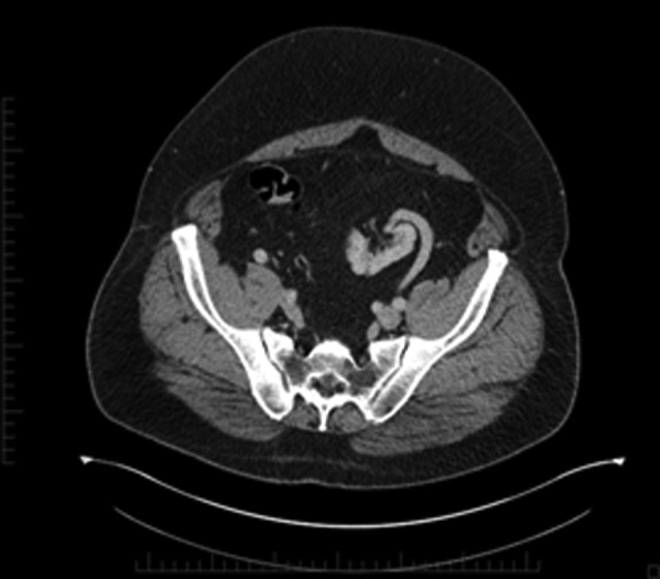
Case 1—CT urogram; axial image showing the medial location of a congenital pelvic kidney, which is rotated with renal vein in the lateral position.
